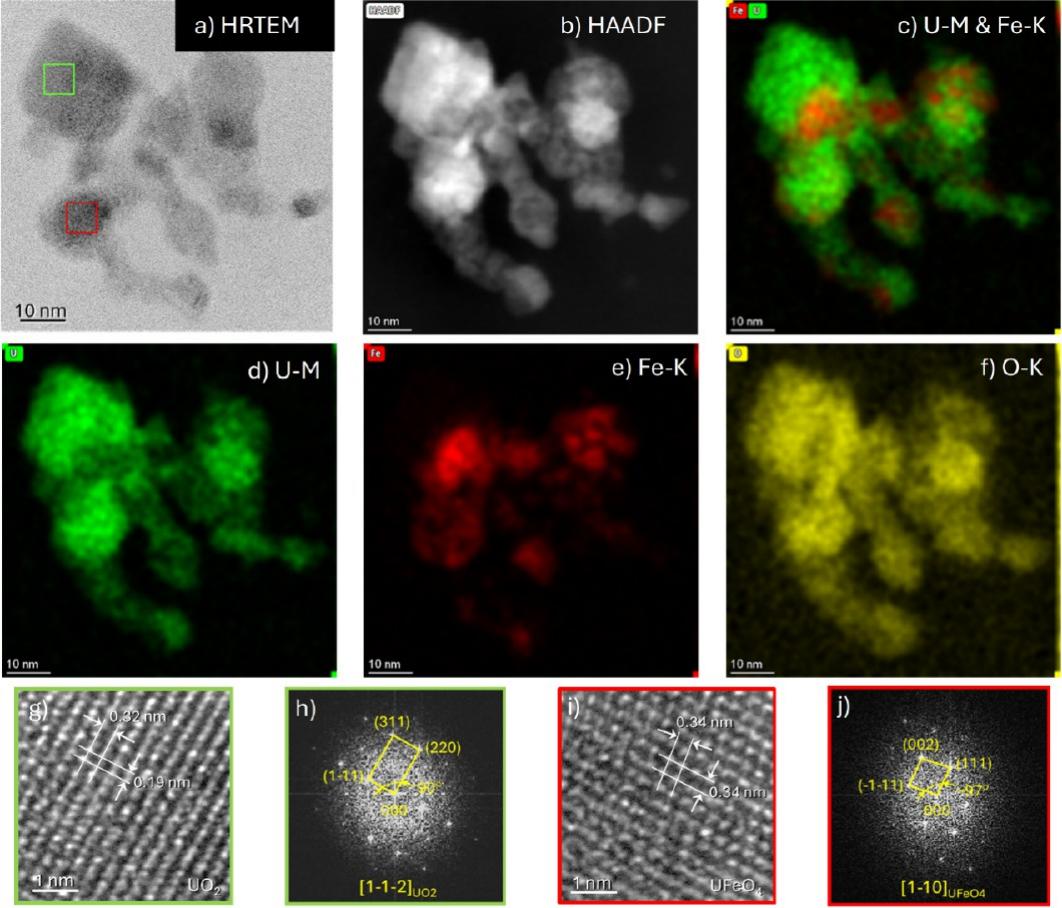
High-resolution TEM analysis and X-ray EDS
